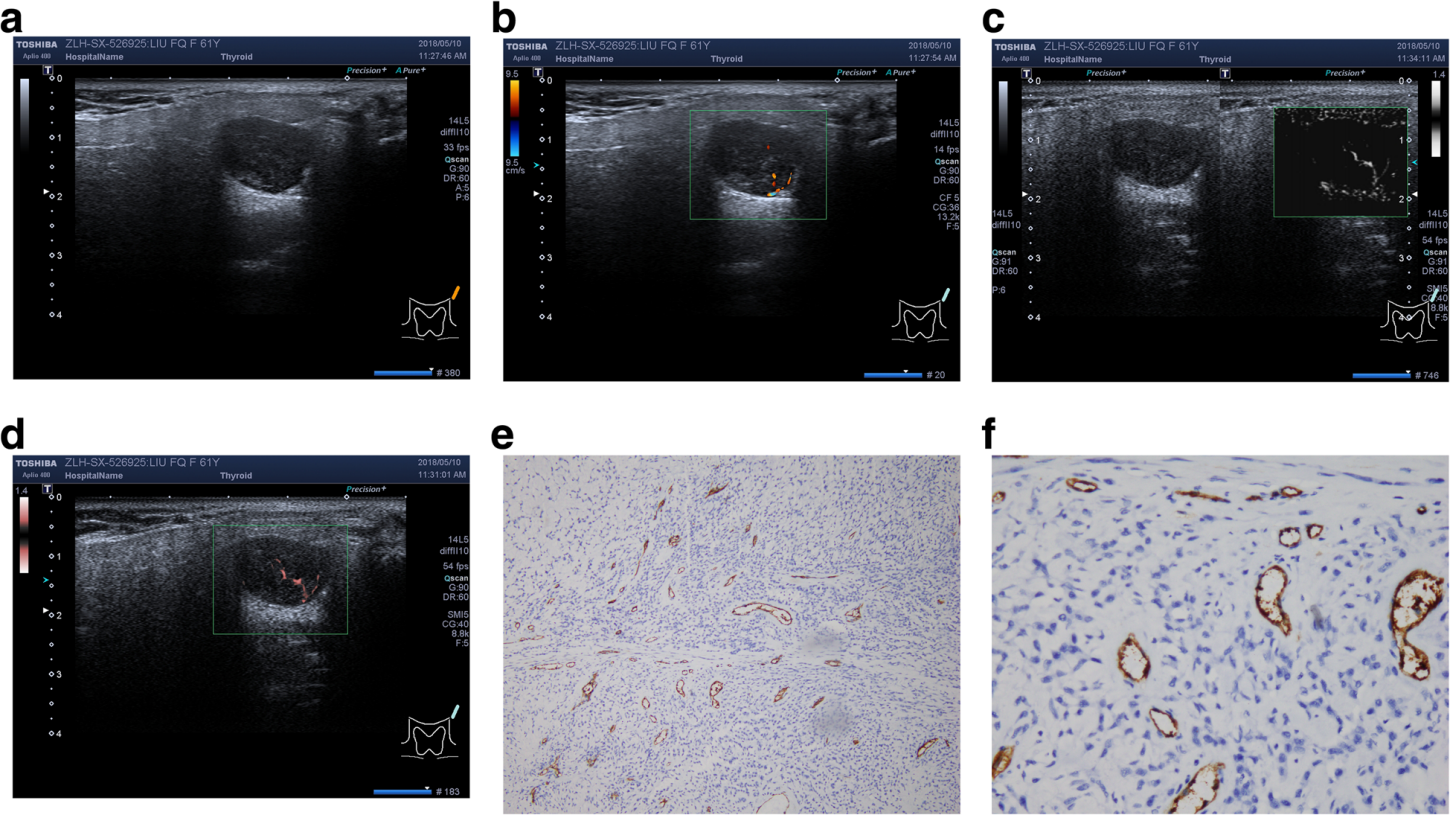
Represent PA case from right parotid gland. a. conventional ultrasound image; b. CDFI: tiny punctate blood flow, Alder 0;c. mSMI and d. cSMI: short cosh or rod-like blood flow, central distribution, Alder 2; VI by cSMI: 1.4; e. MVD by IHC (× 100); f. MVD by IHC: 18 (× 400)
